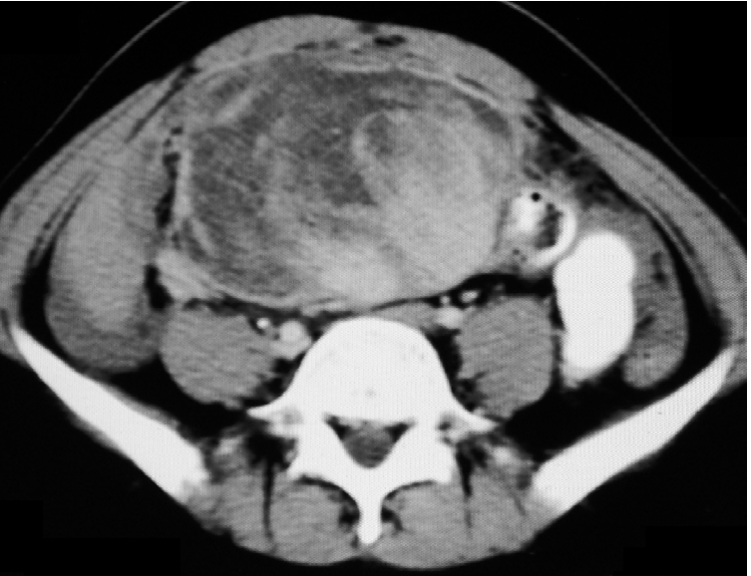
Contrast enhanced CT (CECT) scan of the abdomen done in November 2004 after 6 cycles of ifosfamide and epirubicin combination chemotherapy showing a 10 × 8 cm sized heterogeneously enhancing mesenteric mass.
